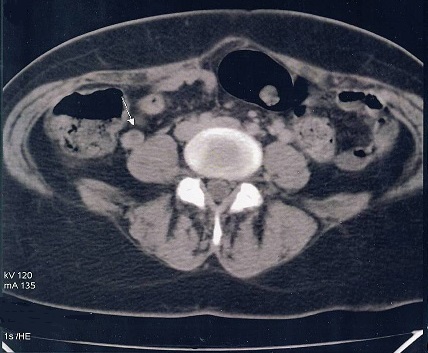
Thrombosis of the right ovarian vein showed on spiral CT
